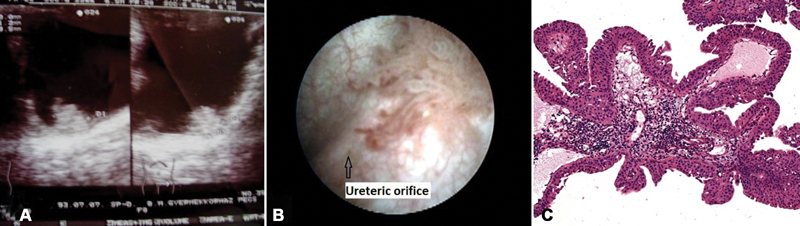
On ultrasound scan, a 15 × 15 mm, solid, hyperreflective, solitary lesion was detected in the urinary bladder next to the orifice of the left ureter. (
